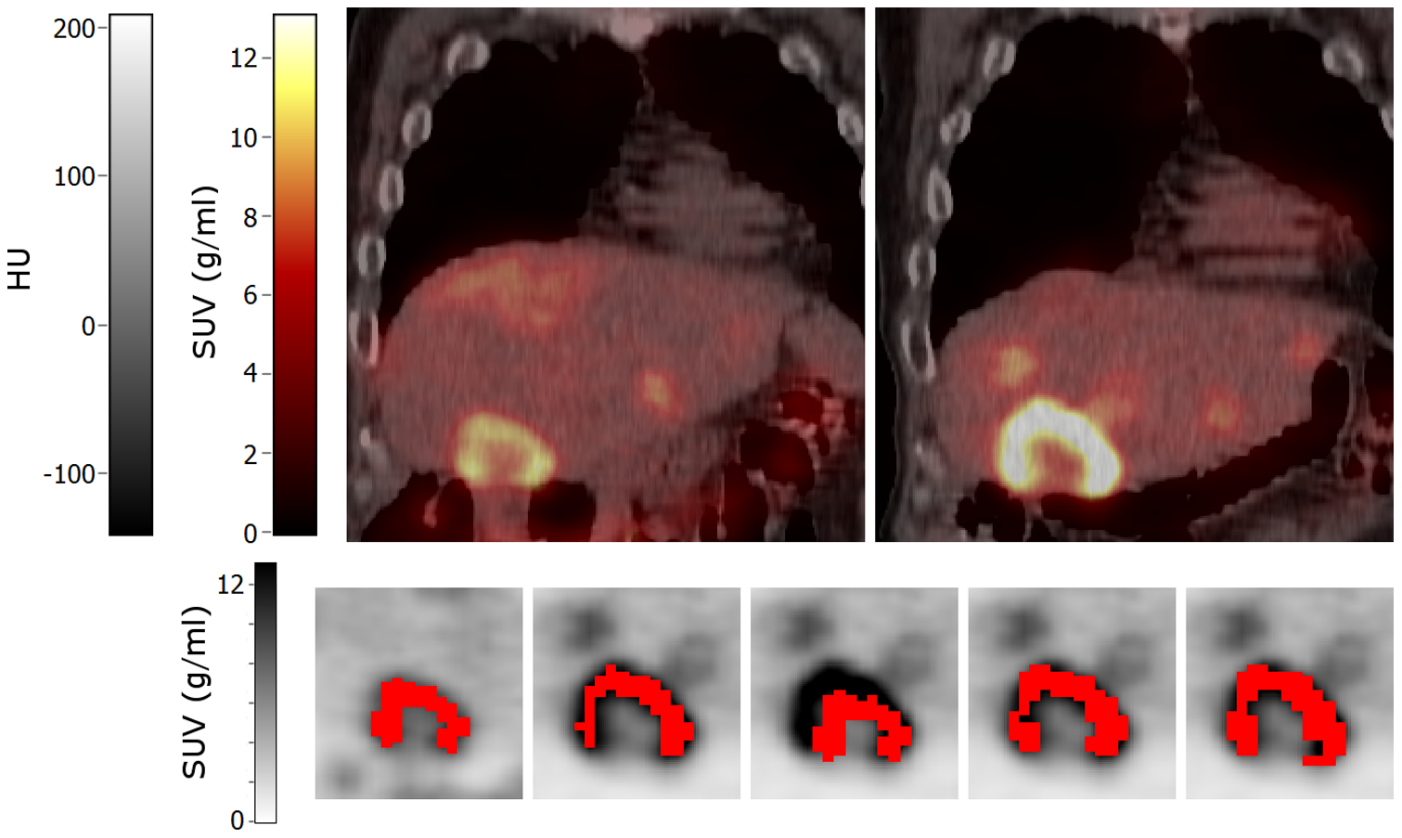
Coronal images of a patient with a liver metastasis that showed an increased metabolically active tumour volume. Top row: baseline (left) and late response (right) PET/CT images. Bottom row: volumes of interest (shown in red) projected onto the baseline (first image) and late response scans (other images) that were obtained using (from left to right): A50 defined on baseline scan, A50 defined on late response scan, and local rigid PET, non-rigid PET and non-rigid CTPET image registration. All images are shown using the same colour scales. Abbreviations: SUV, standardized uptake values; HU, hounsfield units; A50, 3 dimensional (semi-)automatic isocontour method at 50% of the maximum pixel value that corrects for local background.
